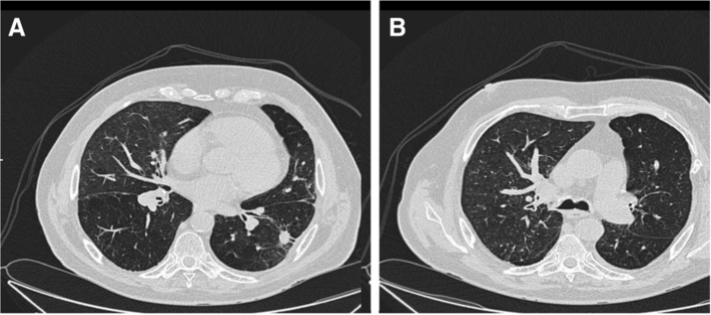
Follow-up CT after 12 month. Chest-CT one year after SBRT showing the residual mass in the left lower lobe (A), and hypoventilation changes in the right upper lobe (B).
